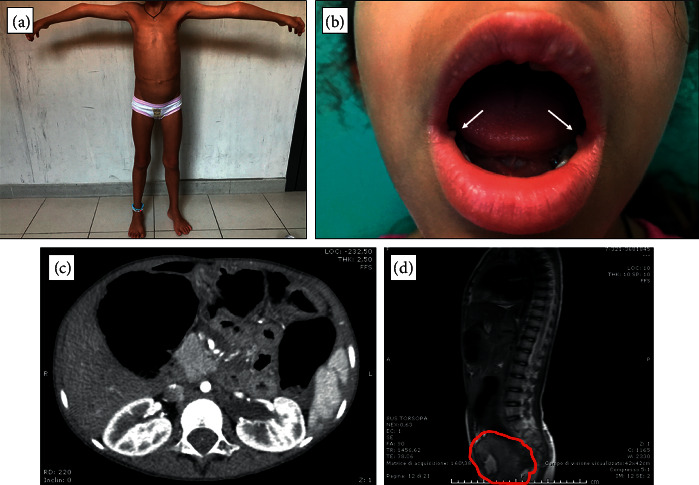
Nonendocrine features present in our patient. (a) Marfanoid habitus with long arms and long legs with respect to the trunk and skeletal abnormalities. (b) Bilateral mucosal neuromas of the mouth as indicated by the 2 arrows. (c) CT cross section of the abdomen showing the megacolon. (d) MRI sagittal section of the pelvic plexiform neurofibroma.
